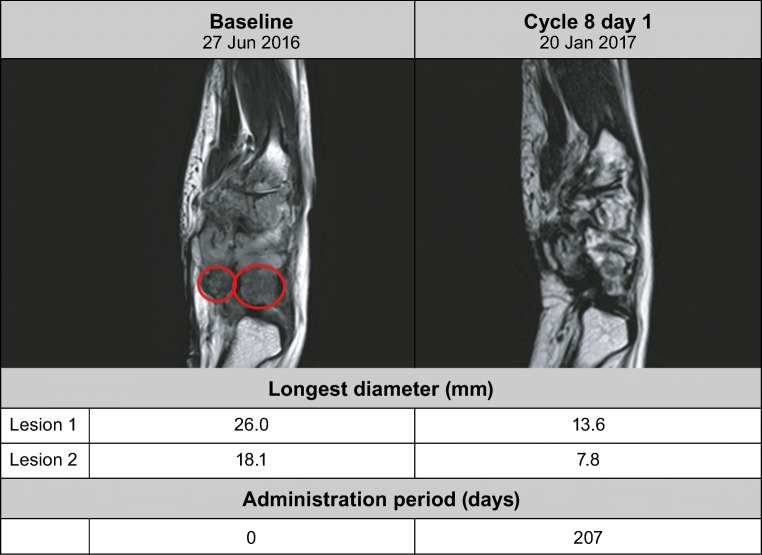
Longest diameter for right wrist synovial cavity by magnetic resonance imaging
